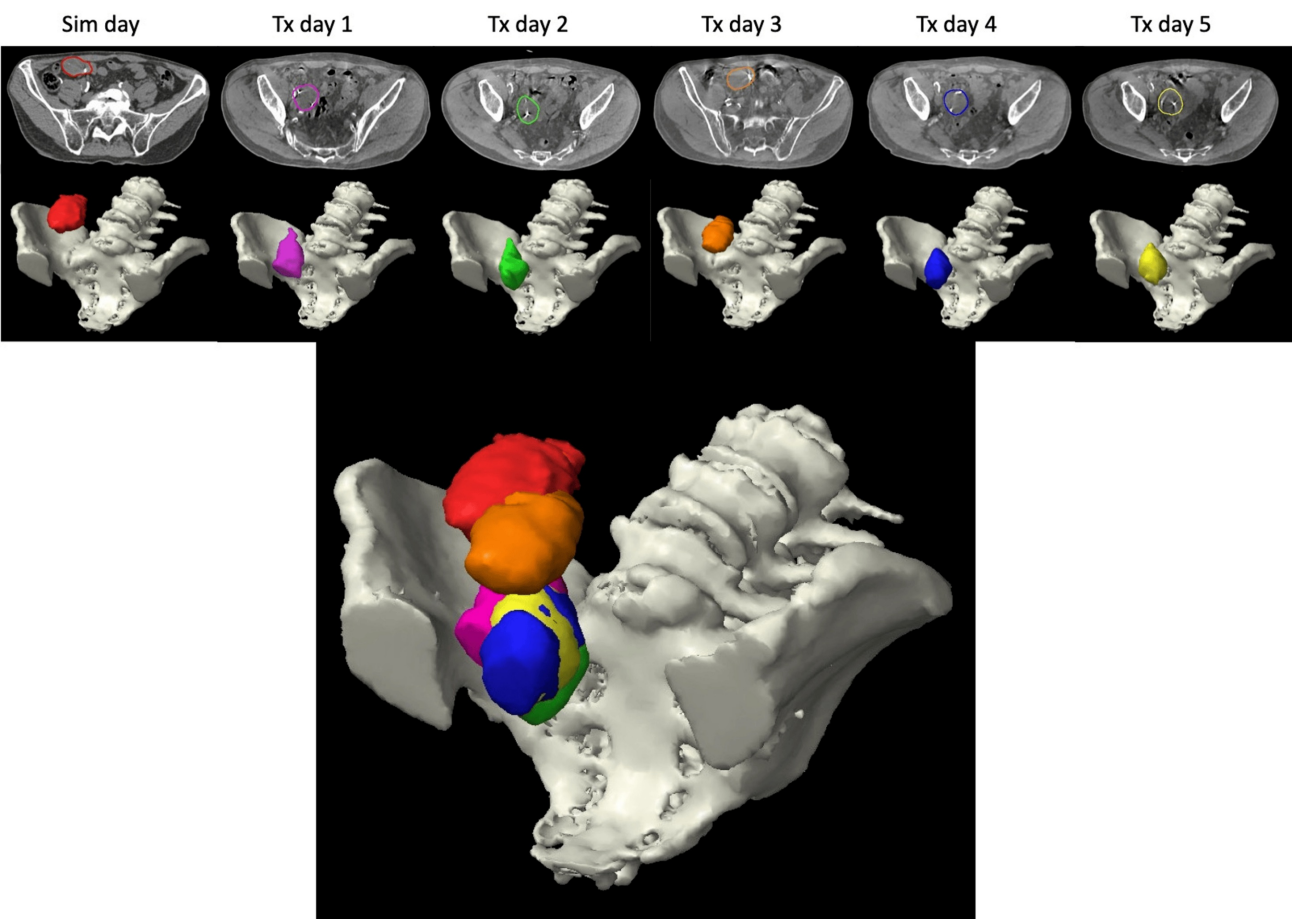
Daily GTVs Daily GTVs re-contoured based on CBCT, compared to initial GTV based on CT sim. Red: CT sim. Pink: treatment day one. Green: treatment day two. Orange: treatment day three. Blue: treatment day four. Yellow: treatment day five CBCT: cone beam computed tomography; GTV: gross tumor volume
